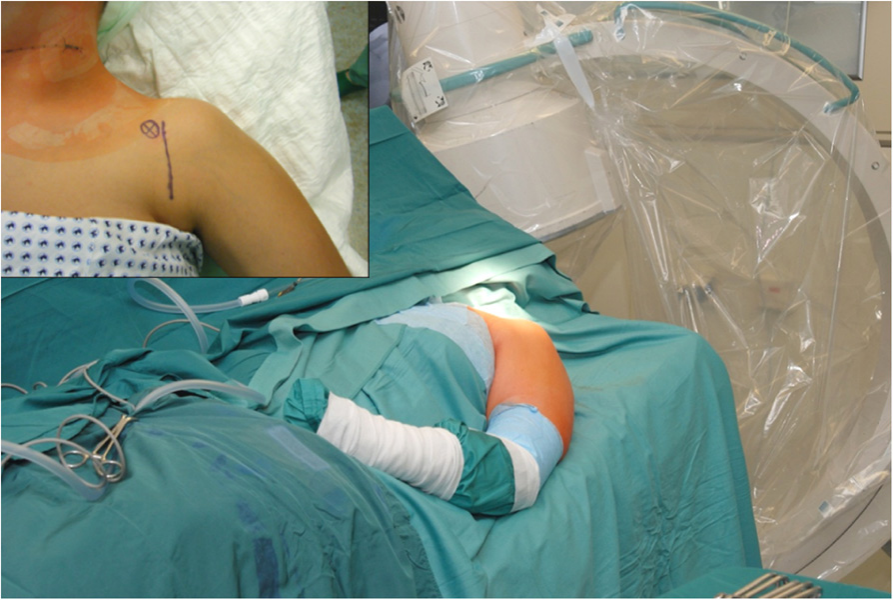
The Semi-Beach-chair Position Used for the Operation. The position must allow true anteroposterior- and axial-view x-rays. The image intensifier is seen in the back. The coracoid process is marked with an X. The skin incision is just lateral to it (insert)
